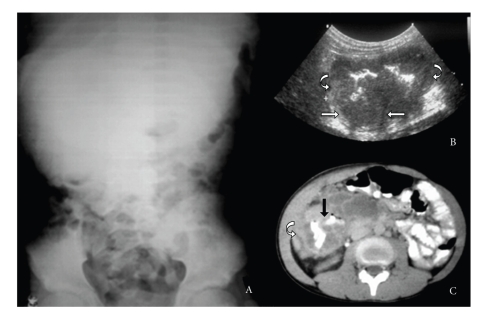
Abdominal X-ray (A) showing a soft-tissue mass with no calcification, on the right flank and mesogastrium, with inferior deviation of the bowel. Abdominal US (B) demonstrates a hypoechoic stenosing mass (curved arrow) measuring about 7.7 centimeters at greatest diameter, in the right colon. Enlarged mesenteric lymph nodes were also identified (arrows). CT of the abdomen (C) confirmed the presence of an infiltrating tumor, slightly enhanced by the contrast media (curved arrow), extending from the cecum to the hepatic flexure on the right colon wall, causing bowel lumen reduction and irregularity (black arrow). The adjacent meso and abdominal wall were also involved.
